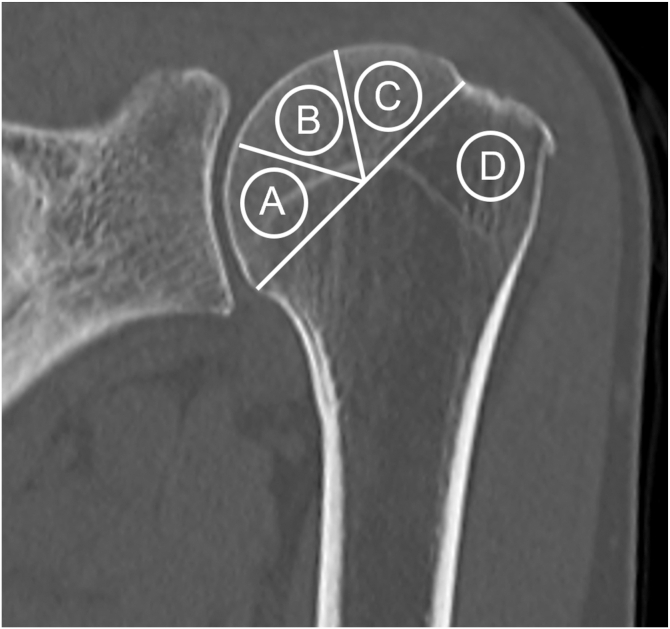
ROI in the proximal humerus. Four ROIs were defined on the coronal CT images: the medial, central, lateral, and greater tuberosity, which were measured within a standardized circular ROI (50 mm^2^) at each location. *ROIs*, regions of interest; *CT*, computed tomography.
